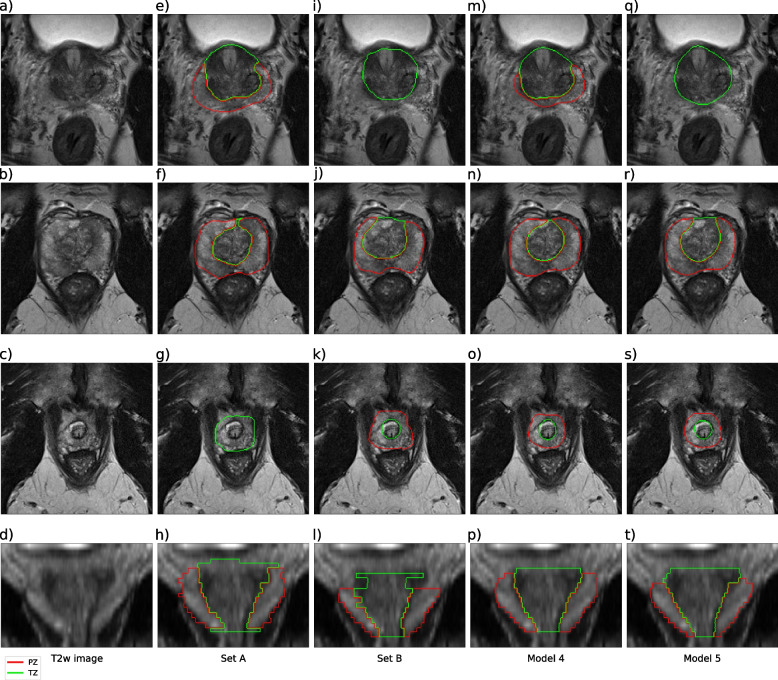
Examples of manual and automatic segmentation. The figure shows segmentations of the transition zone (TZ) and peripheral zone (PZ) of a case from PROSTATEx that is part of the test set for models 4 and 5. Axial images of the prostate base, mid, and apex sections are shown in rows 1, 2, and 3, respectively. A coronal image of the prostate is shown in row 4. a–d T2-weighted images, the rest are segmentations from set A (e–h), set B (i–l), model 4 (m–p), and model 5 (q–t)
